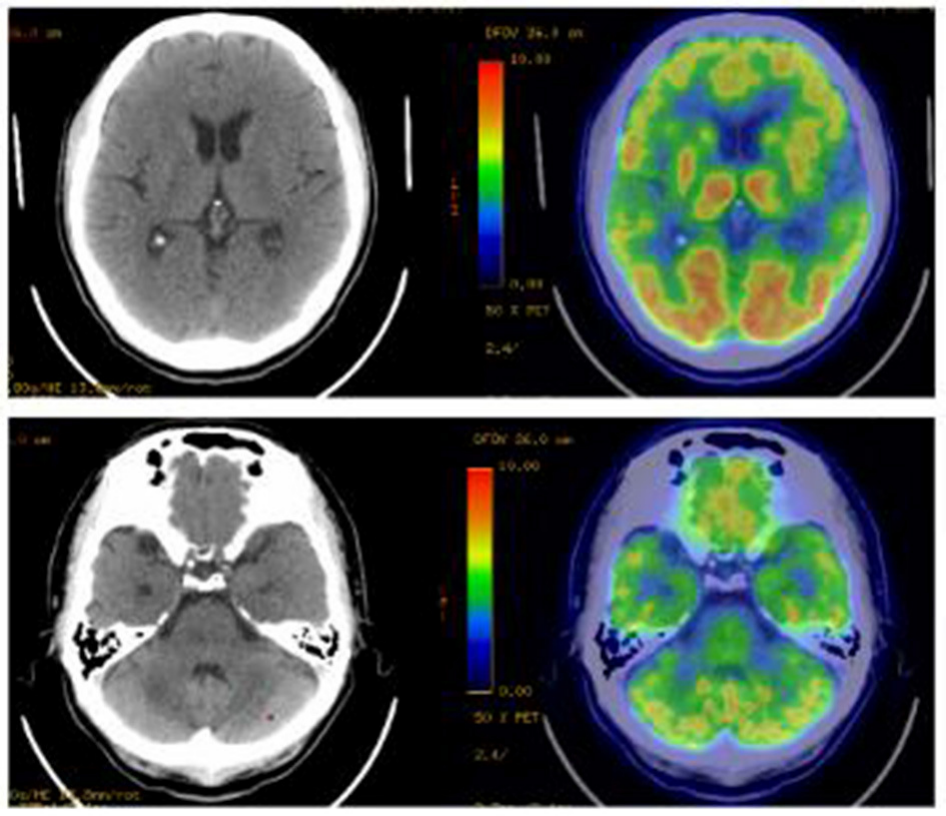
PET-CT imaging. Bilateral temporal lobe and cerebellar reduced fluorodeoxyglucose metabolism level. PET-CT, Positron Emission Tomography and Computed Tomography.
